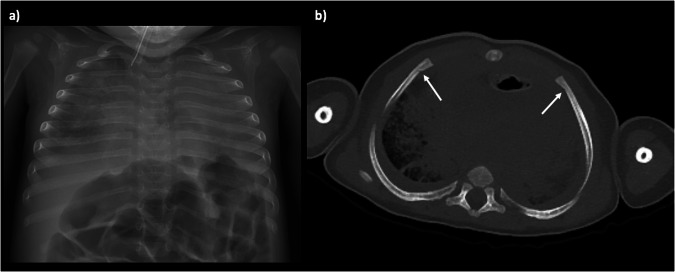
Postmortem chest radiograph (a) and axial PMCT image through the thorax (b) in the same infant demonstrating bilateral symmetrical greenstick rib fractures of the ribs on PMCT (arrows), around 2 cm posterior to the costochondral junction caused by resuscitation, which are difficult to see on plain radiographs, but in a typical expected location
